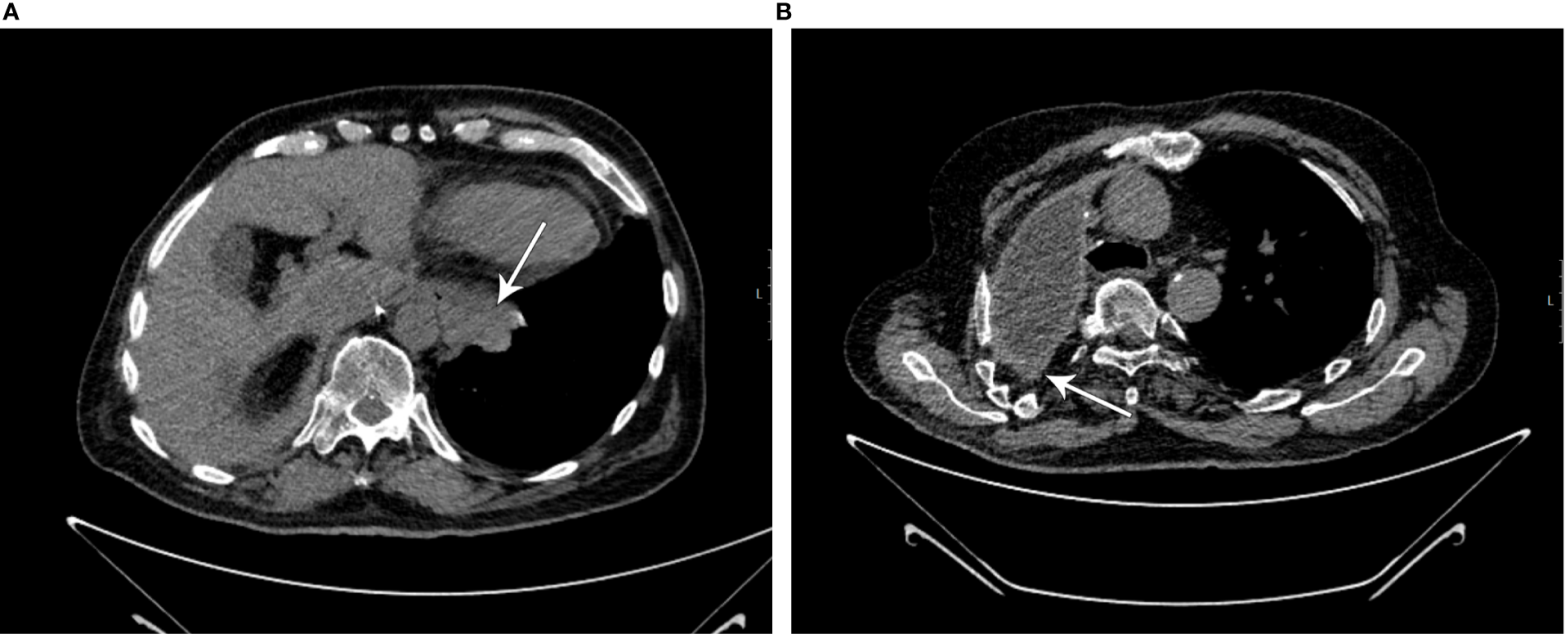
(A, B) CT scans showing progression on second-line therapy (marked by white arrows; initial scans for pembrolizumab treatment).
